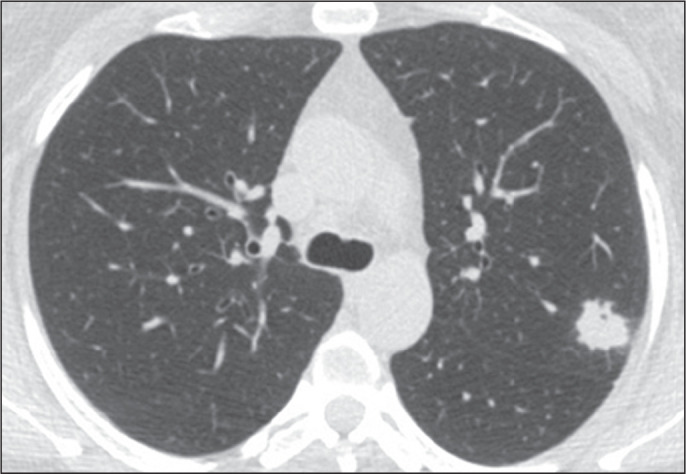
CT of an asymptomatic 36-year-old woman who tested positive for
